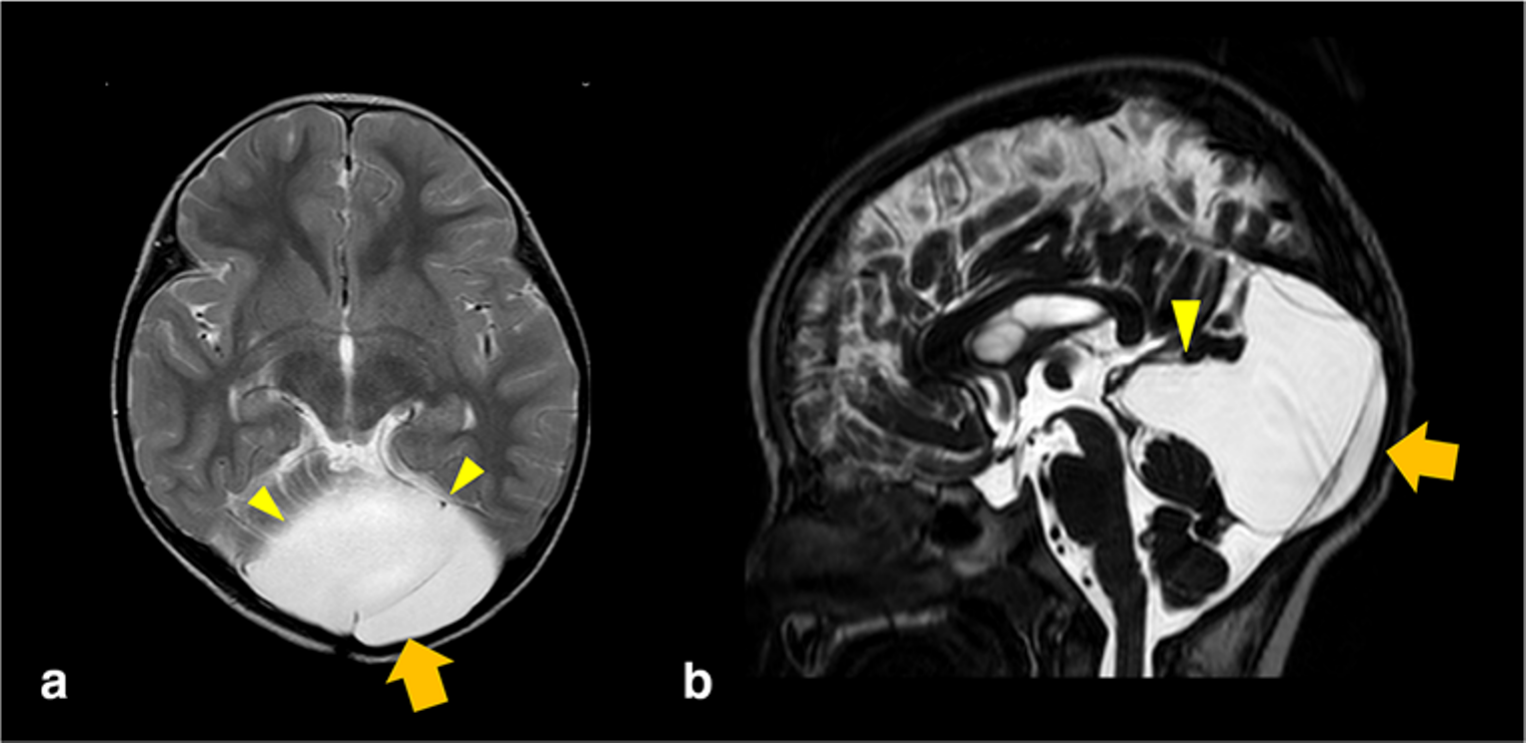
Postoperative MRI scan. a Axial T2-weighted image and b sagittal T2-weighted restored pulse image showing reduction of QAC (small yellow triangle). Rigid neuroendoscope inserted from left side of the posterior bone (large orange arrow)
